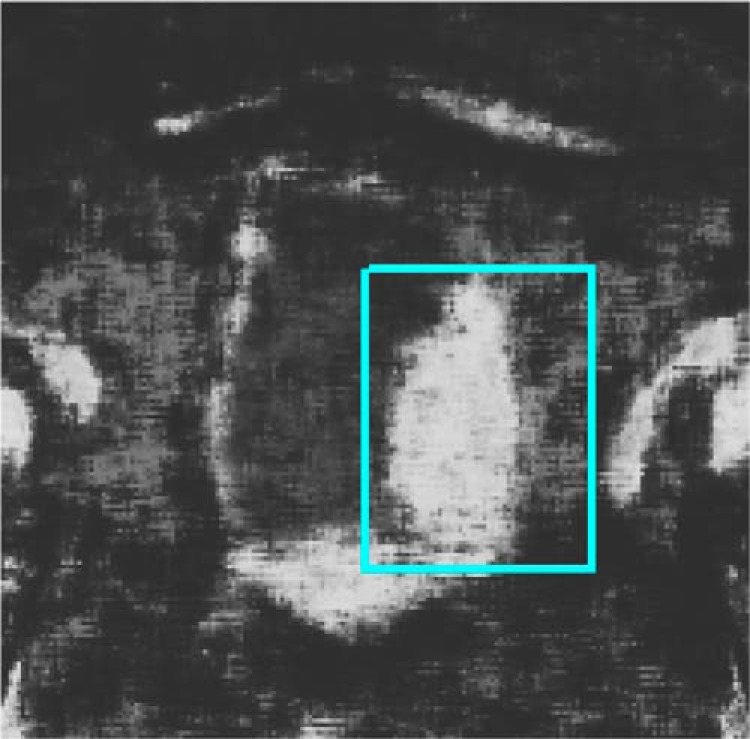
Bladder cancer likelihood map of the CT section shown in Figure 1. Regions that are highly likely to be bladder cancer have higher intensity values. The volume of interest (VOI) that was used for this lesion is shown in blue. For demonstration purposes, the bladder cancer likelihood map was generated in the region around the entire bladder.
